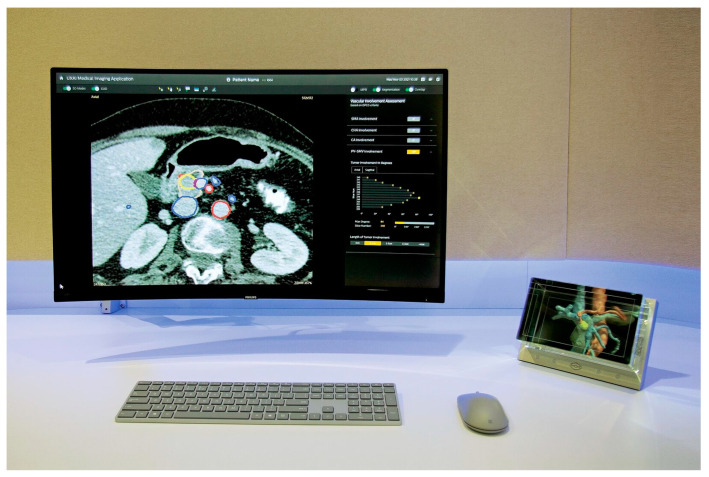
The integrated medical imaging workstation. The integrated medical imaging workstation consisting of a DICOM viewer showing the CT scan, segmentations, and the Looking Glass holographic display. The segmentations including information on tumor vessel contact were reconstructed and displayed on the Looking Glass holographic display.
